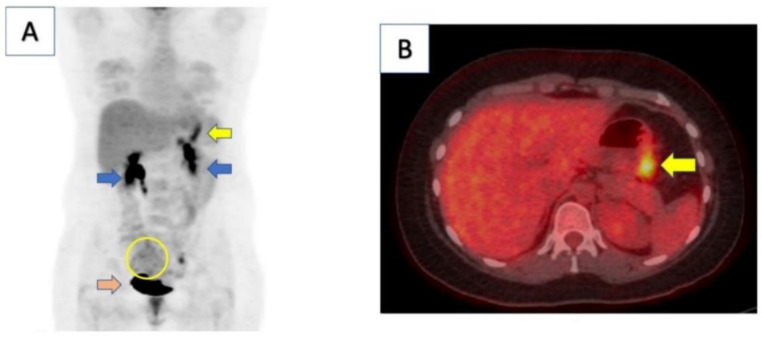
Computed tomography (CT)/positron emission tomography (PET) scan of patient demonstrated moderate focal uptake along gastric body consistent with known malignancy (yellow arrow in panel (A,B)). There was also a large, mildly hypermetabolic right adnexal area with heterogenous uptake, indicating metastatic involvement (yellow circle). Kidneys (blue arrows) and bladder (orange arrow) demonstrate physiologic uptake. Reproduced from [15].
